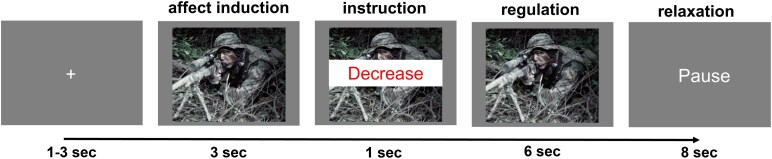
Example of one functional magnetic resonance imaging trial in the downregulate-negative condition. In the behavioural task, instead of a relaxation phase, participants were asked to rate the intensity of their negative feelings on a 7-point Likert scale at the end of each trial.
